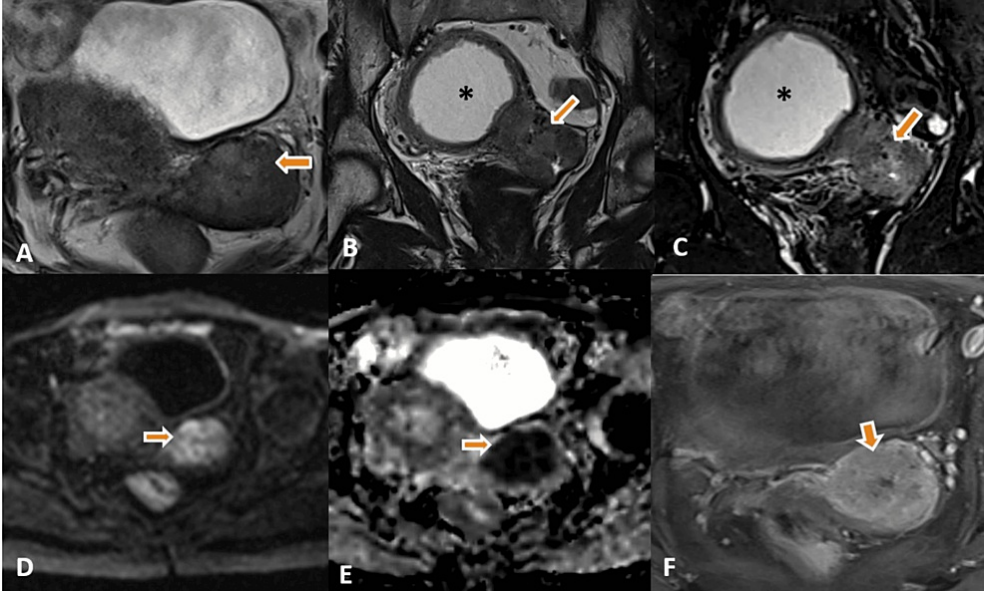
A-F: Axial (A) and coronal (B) T2-weighted and STIR coronal (C) images show endocervical infiltrative heterogeneously high signal intensity lesion involving cervix (thick arrows). Significant cervical stenosis with resultant hydrometra and an enlarged uterus is also seen (B, C asterisk). The lesion shows significant diffusion restriction (D, thick arrow) and a correspondingly low signal on ADC images (E, thick arrow). The axial post-contrast fat-saturated T1-weighted image shows heterogeneous enhancement of the lesion in the cervix (F, thick arrow).
